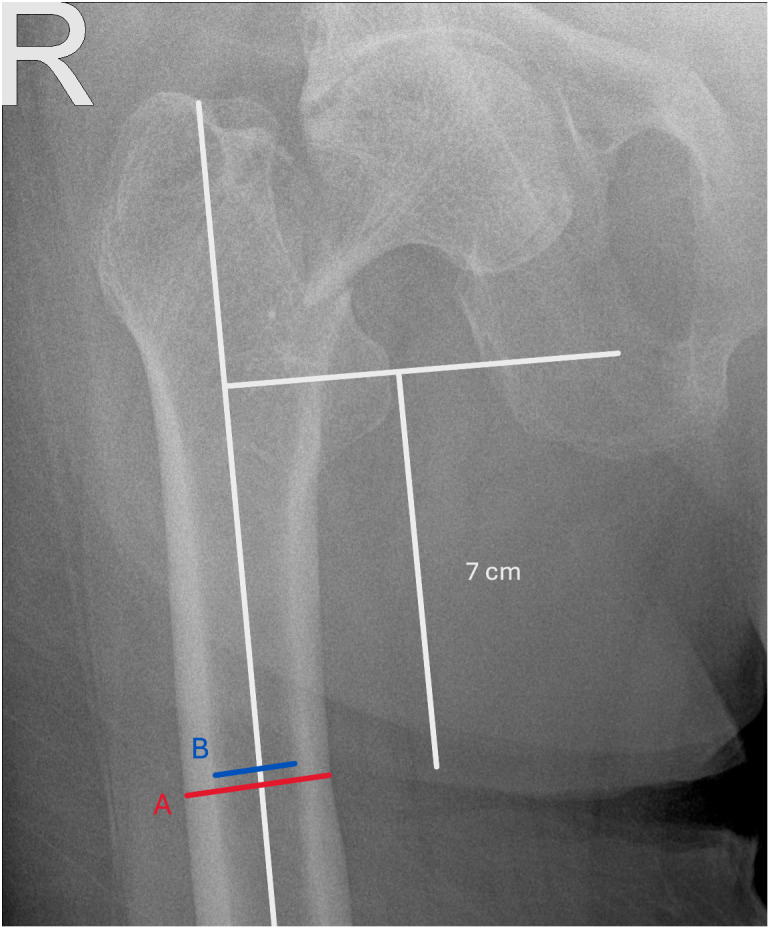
Measurement of the canal-to-bone ratio (CBR) on anteroposterior pelvic radiographs. The outer cortical diameter (red line, A) and the intramedullary canal diameter (blue line, B) were obtained at a standardized level located 7 cm distal to the lesser trochanter. The CBR was then calculated as the ratio of B to A.
